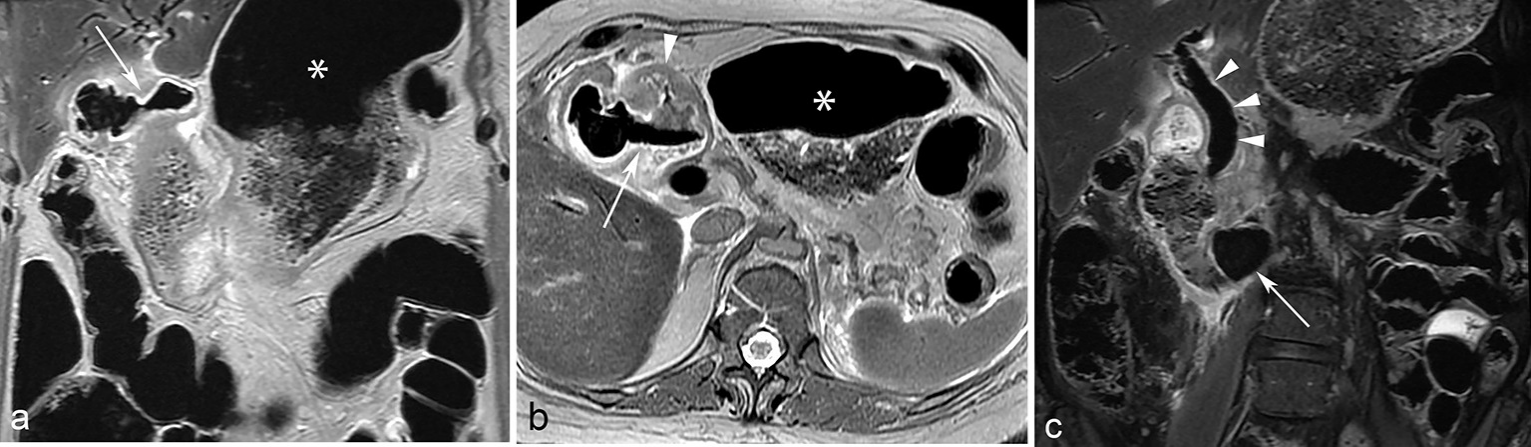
Post-mortem unenhanced MRI in case of Bouveret’s syndrome. Coronal (a) and axial (b) *T*_2_ weighted images through the level of the gallbladder fossa clearly demonstrate a widely patent gas-filled fistula (arrow) extending from the gallbladder to the adjacent duodenal bulb (arrow), just beyond the pylorus (arrowhead). The fistula is peripherally lined with hyperintense fluid. Debris can be seen filling and distending the stomach (asterisk). Coronal *T*_2_ weighted fat suppressed (c) image demonstrates a large signal void representing the obstructing duodenal gallstone (arrow), partially surrounded by hyperintense fluid in the dilated proximal duodenum. The extrahepatic bile duct (arrowheads) is dilated and gas-filled.
